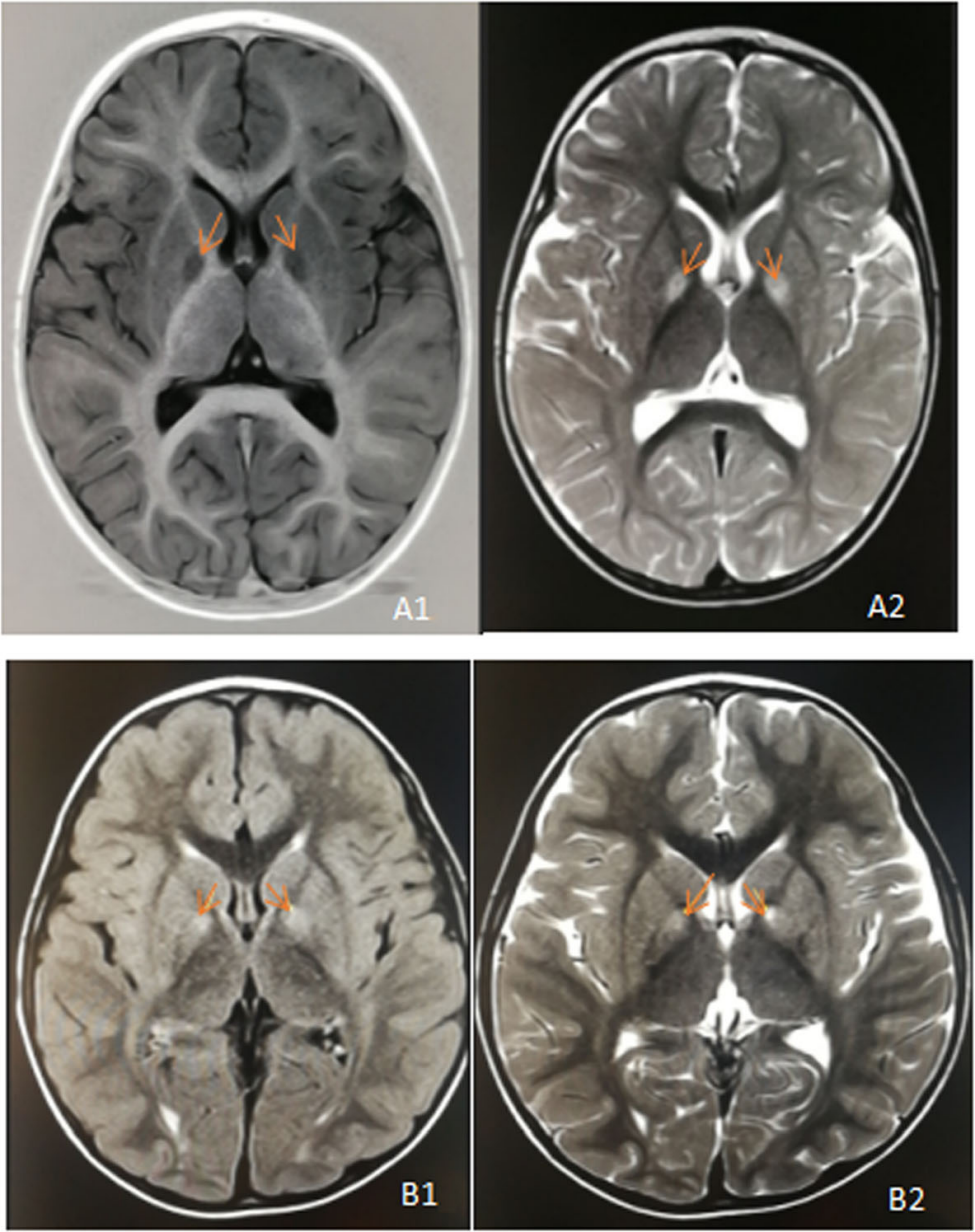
a Brain MRI of patient 1 showed bilateral globus pallidus swelling and abnormal signal (as shown by arrow), T1 W1 (A1) showed low signal and T2 W1 (A2) showed high signal; b Brain MRI of patient 2 showed a little patchy abnormal signal of bilateral globus pallidus (as shown by arrow), small patchy shadow of left globus pallidus adjacent to anterior limb of internal capsule showed slightly obvious, and both T2WI-flair(B1) and T2WI(B2)showed high signal
